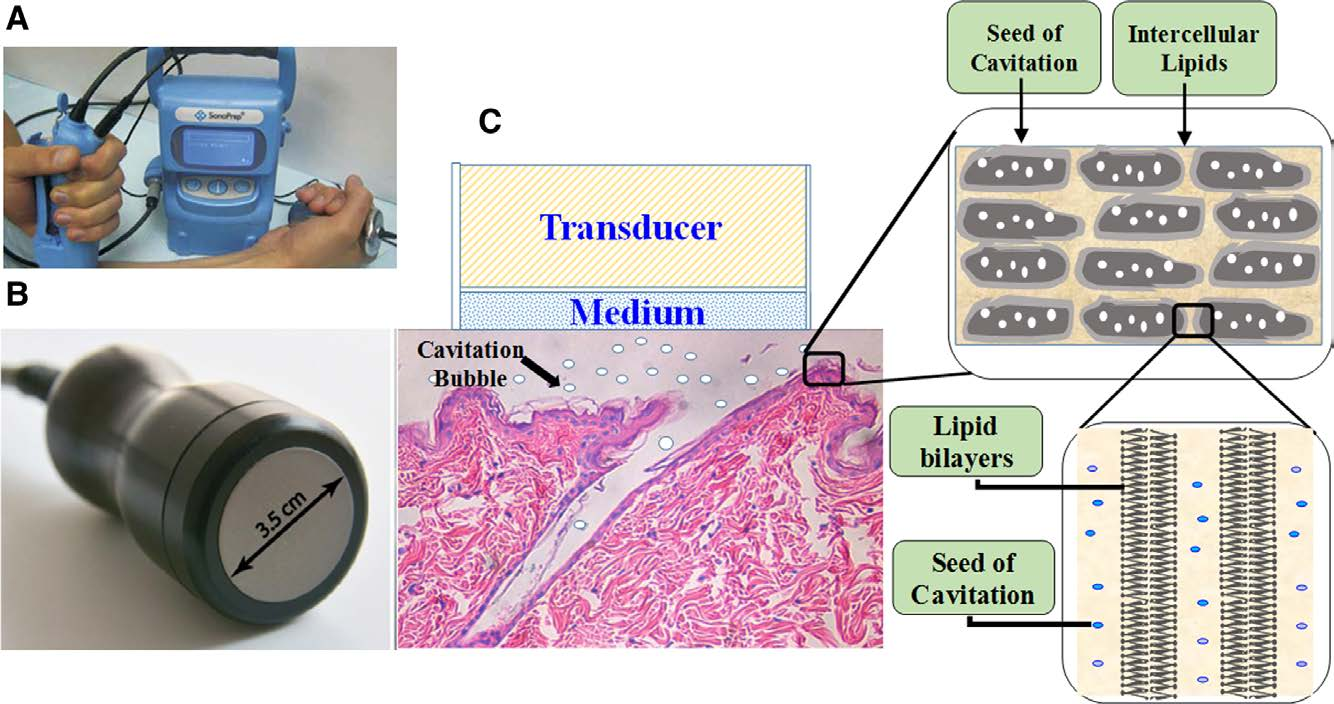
Sonophoresis for transdermal permeation. (A) Low-frequency ultrasound treatment in a clinical setting with the SonoPrep® device (Echo Therapeutics, Franklin, MA). (B) Ultrasound transducer, 3.5-cm diameter. (C) Mechanistic overview of sonophoresis-facilitated drug delivery
